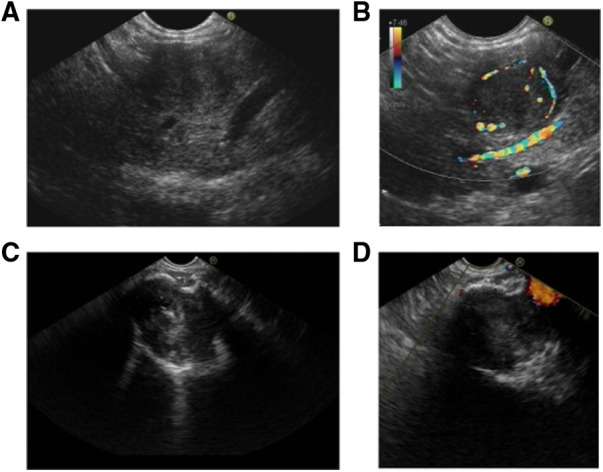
Characteristics of pNET and SPT in EUS. (A,B) pNET in a 54-year-old man with no physical discomfort. A homogeneous and hypoechoic lesion with a clear border was found in the pancreatic body. CDFI indicated the distinct peritumoral blood flow. (C,D) SPT identified in a 36-year-old woman during physical examination. A heterogeneous and hypoechoic lesion with a clear border was identified in the pancreatic neck. CDFI showed no significant blood flow signal. Hyperechoic calcification was observed in the lesion. pNET, pancreatic neuroendocrine tumor; EUS, endoscopic ultrasound; SPT, solid pseudopapillary tumor; CDFI, color Doppler flow imaging.
